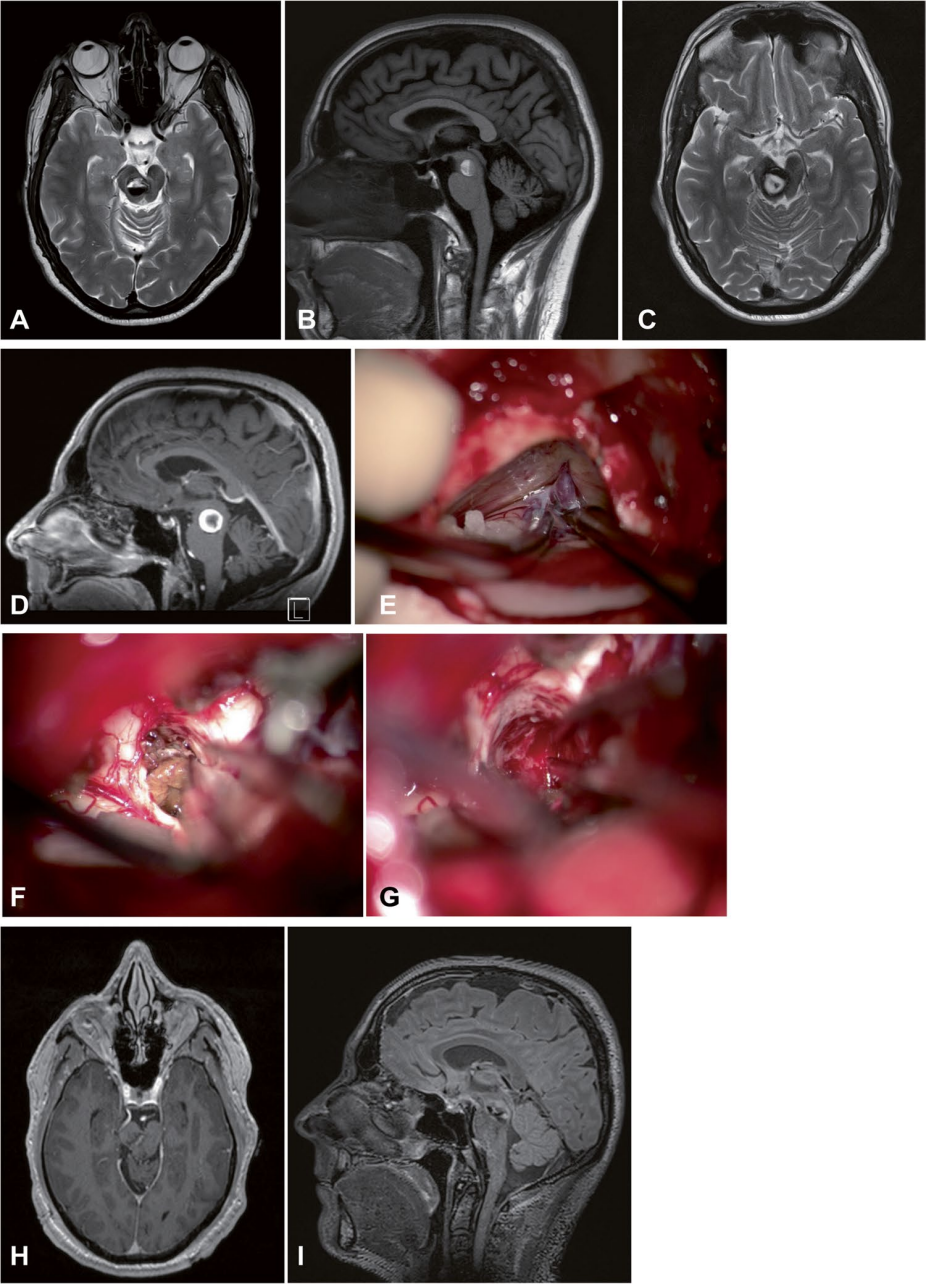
A 56-year-old man presented with diplopia, abduction weakness of the right eye, dysarthria, weakness in both hands. He was referred to our department after the first diagnosis of BSCM bleeding in another hospital. The MRI scan revealed a 19 × 18 × 13 mm BSCM in the ponto-mesencephalon (A and B). The BSCM score was grade VI (size = 1, mRS = 2, hemorrhage event = 1, age = 1, and no midline crossing = 1), and conservative treatment was suggested. Two weeks later, the patient had another BSCM bleeding without an increase in lesion size or deterioration of neurological deficits (C and D). The BSCM score was grade VII. Surgical resection of BSCM was performed with a retrosigmoid approach (E, F, and G). Postoperative MRI confirmed the total resection (H and I). The patient was neurologically intact at the last follow-up one and a half years later
